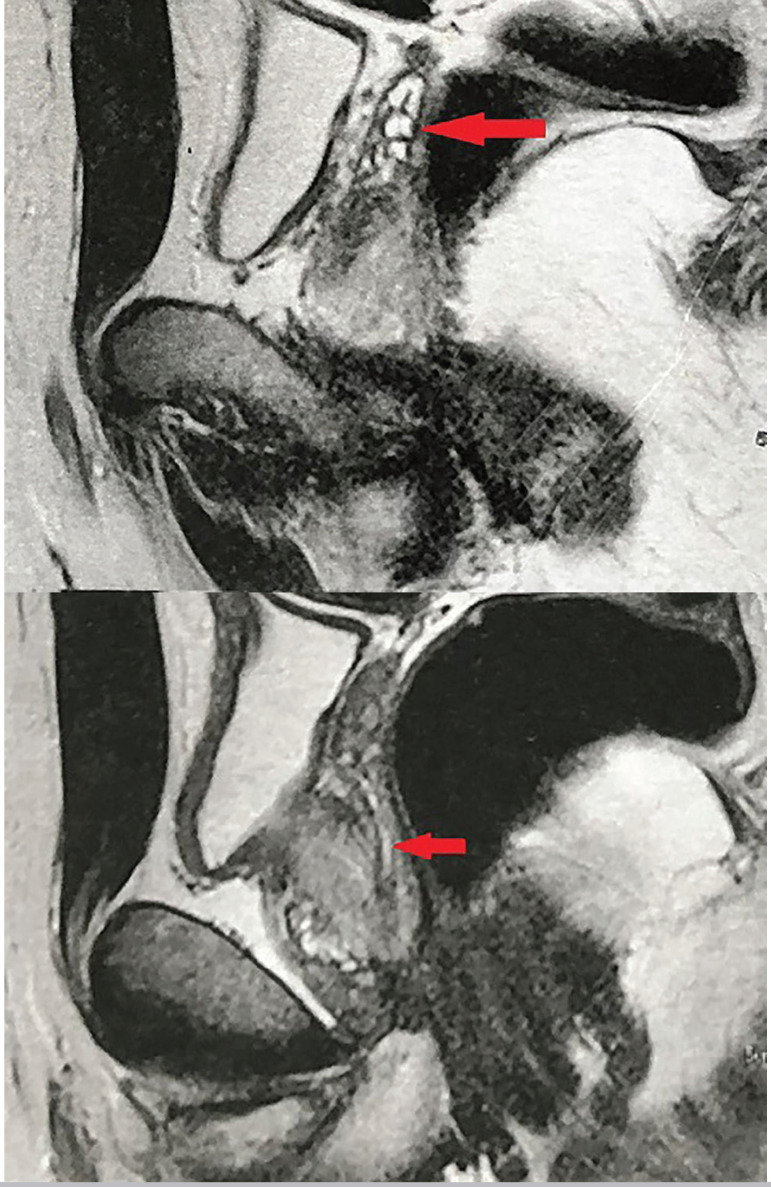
MRI. Dilation of the right SV and ED with no signs of inflammatory, neoplastic or cystic lesions in the prostate and SVs
